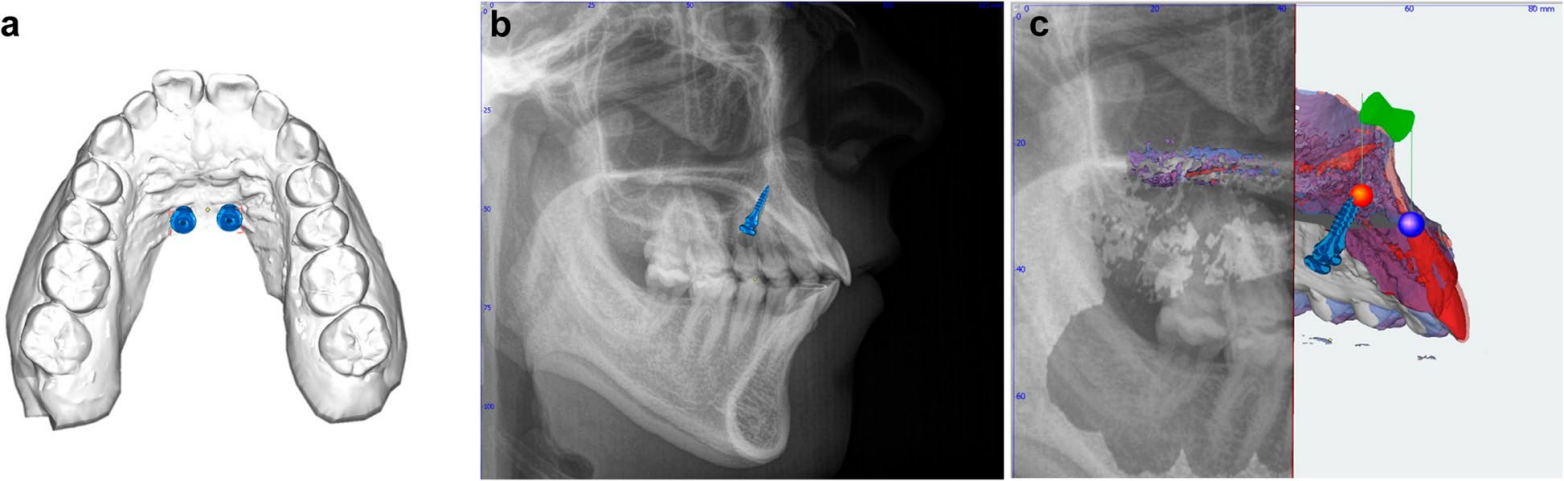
Sequence of insertion and measurements. Placement according to clinical guidelines (a) correction of position and angle using the lateral cephalogram, followed by measurement of the distance between miniscrews and incisors (b) application of the CBCT for measurement of the distances between both implants and central and lateral incisors
